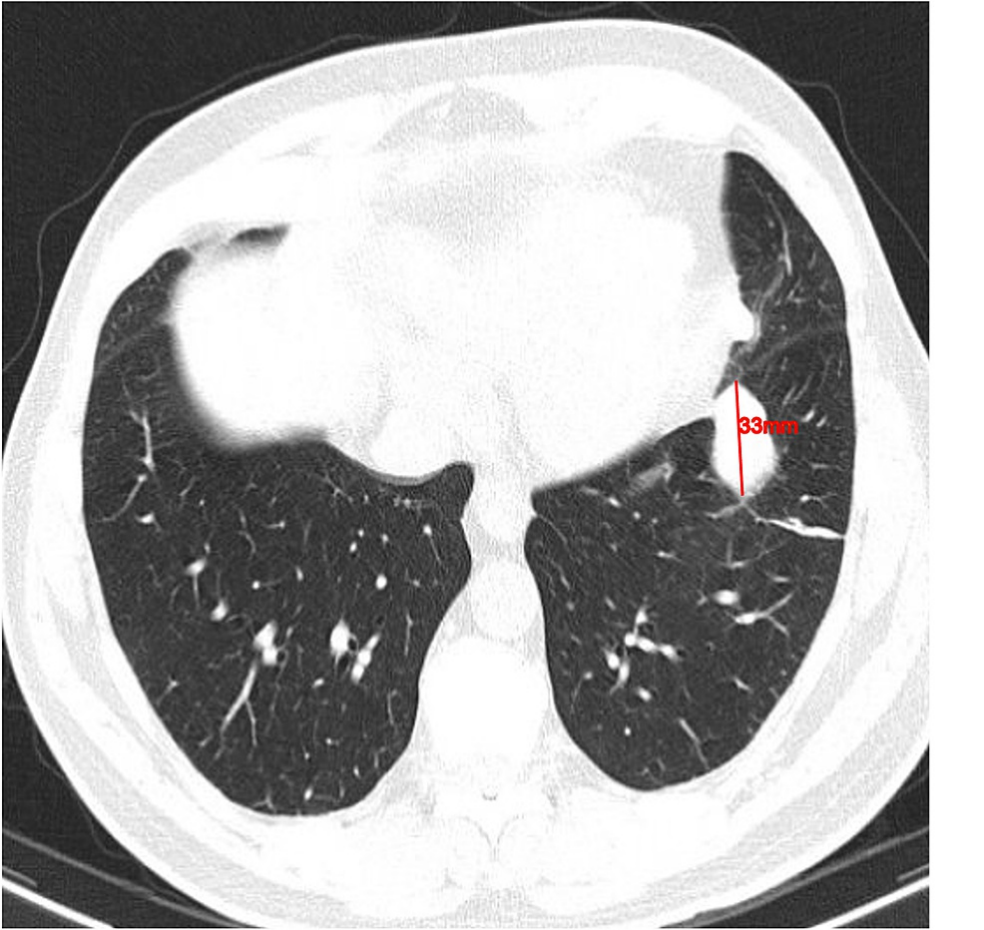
Non-enhanced computed tomography Juxta-pleural thickening, in a nodular shape, of 33 mm longitudinal diameter in the left inferior lobe.
